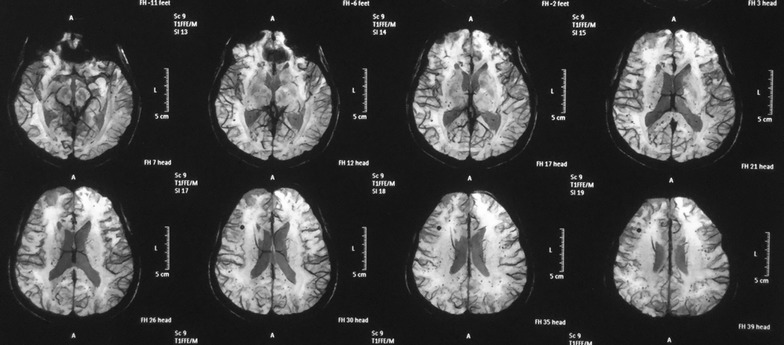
Magnetic resonance imaging of brain. Showing extensive minute haemorrhagic foci throughout the cerebrum
